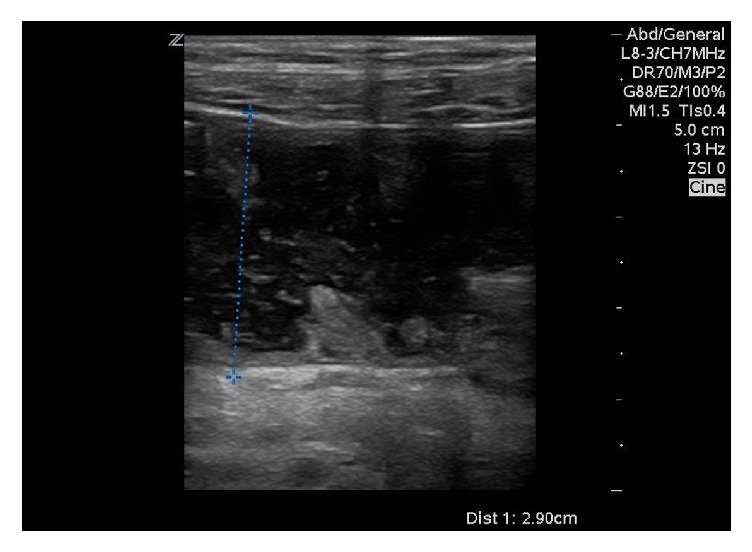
Ultrasound image using a high frequency linear transducer shows a dilated loop of bowel, with a width of 2.9 cm in the left lower quadrant compatible with a small bowel obstruction.
